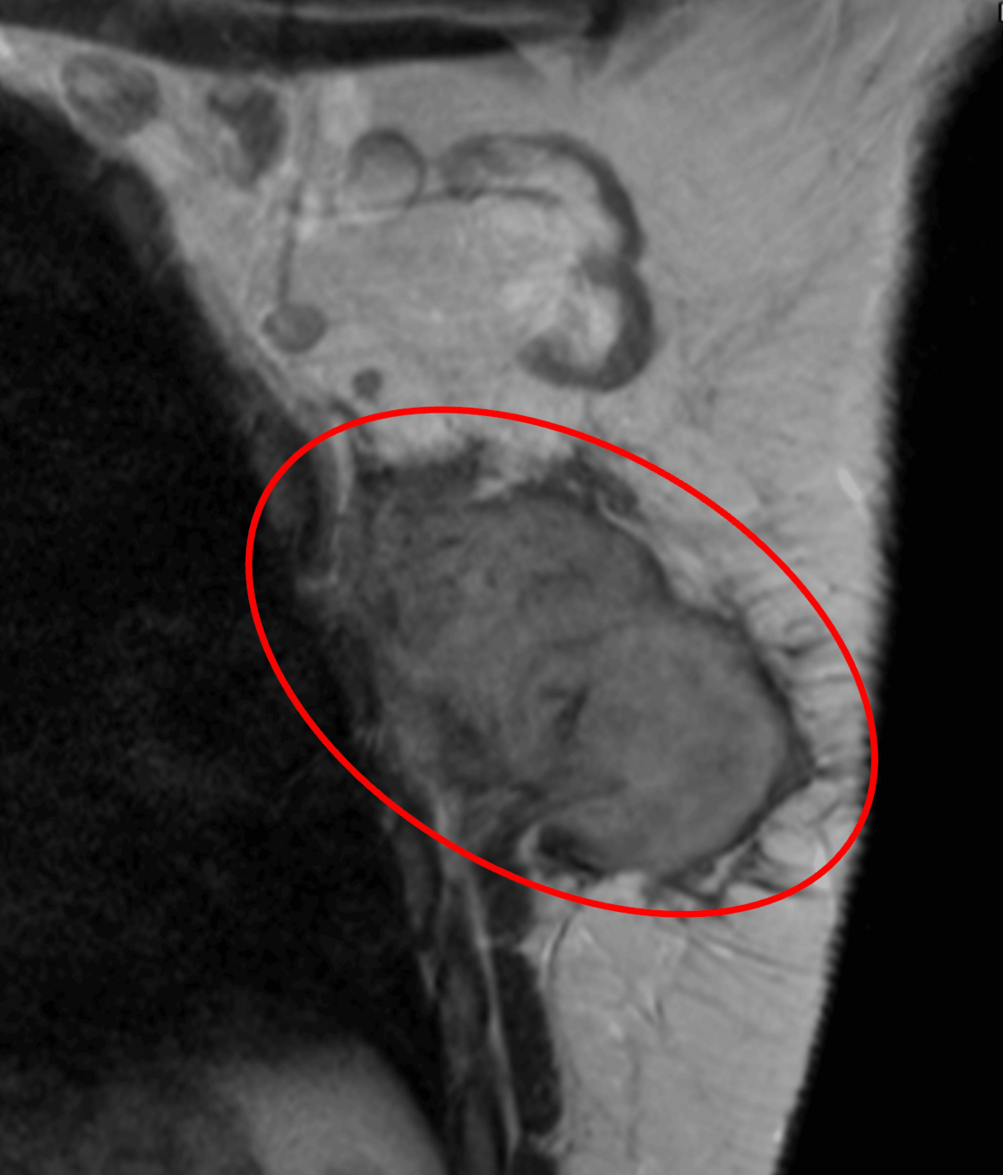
Coronal contrast-enhanced MRI scan of the left breast acquired on 3T Coronal contrast-enhanced MRI scan of the left breast acquired on 3T (9mL gadobutrol) T2-weighted Dixon image (repetition time scan/echo time msec, 3.840/94, section thickness 5 mm) showed heterogeneous progressive internal enhancement of the mass with irregular margins.
